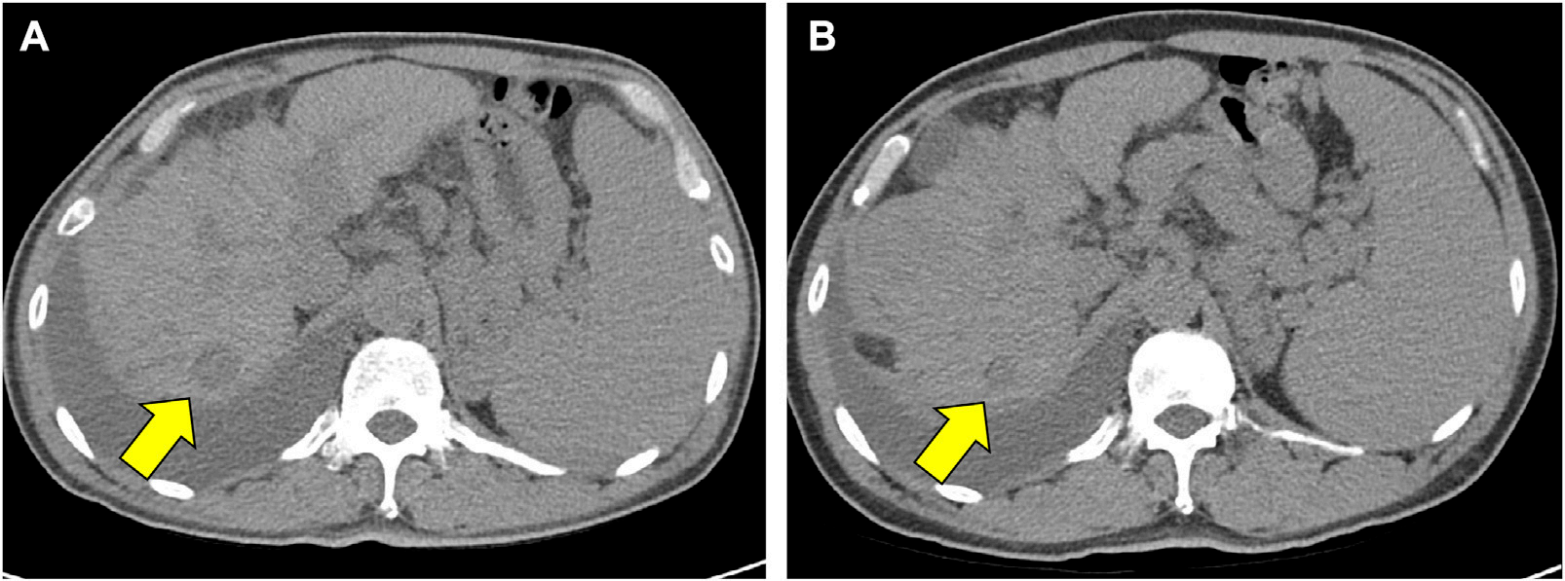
Decrease of tumor size in the CT scan images. (A) Is an abdomen CT image which were scanned on July 23 (B) is a follow-up abdomen CT image which were scanned on December 22. Yellow arrows indicate a decrease in the longest diameter of tumor mass from 2.28 cm in (A), to 1.60 cm in (B). Abbreviations: CT, computed tomography.
